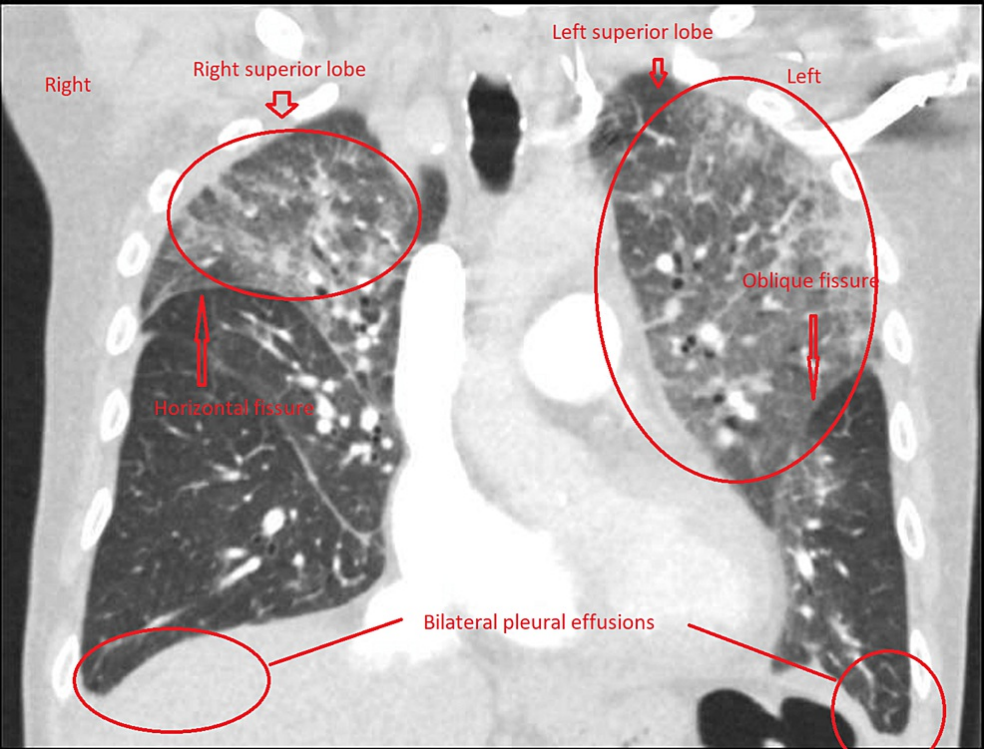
Computed tomography pulmonary angiogram coronal plane. Computed tomography pulmonary angiogram showing pulmonary oedema involving bilateral superior lobes and pleural effusions.
